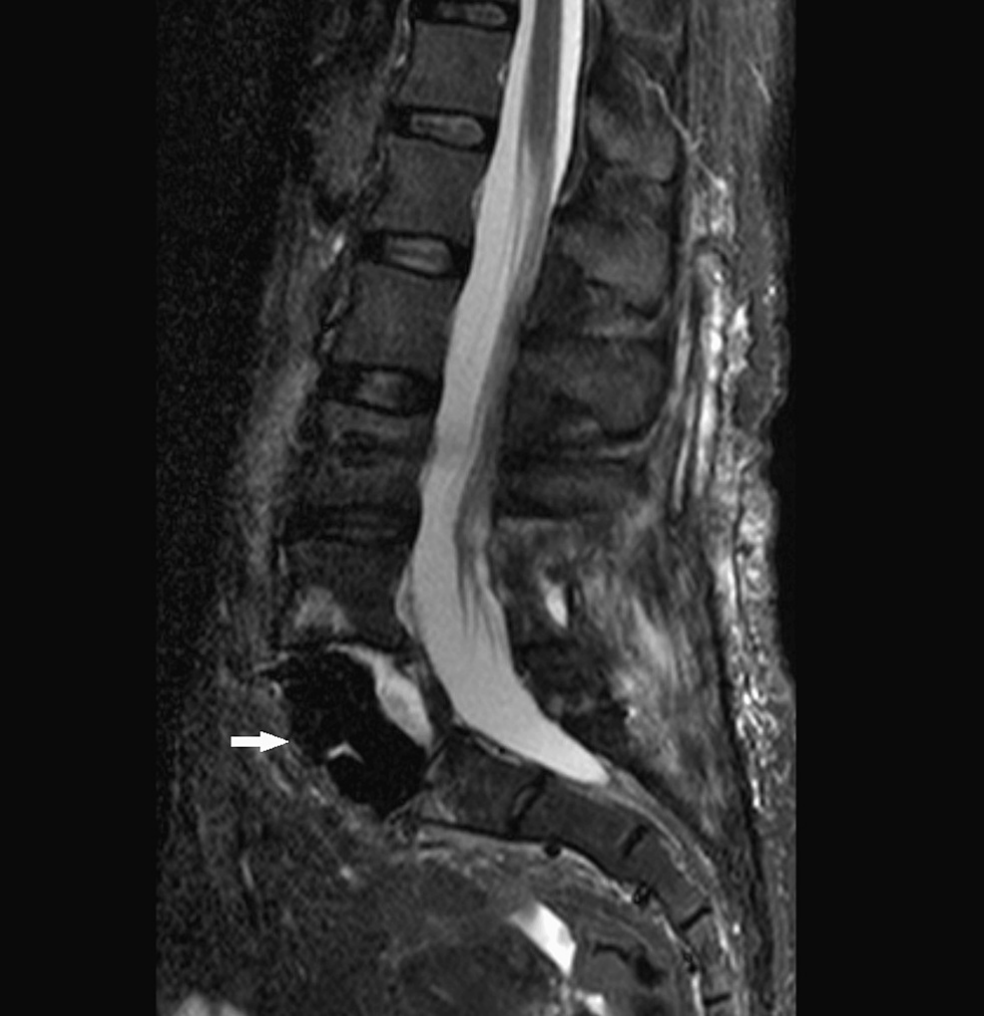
Lumbar MRI after surgery, sagittal T2-STIR. Lumbar MRI showed no persistence of disease on sagittal T2-STIR.
